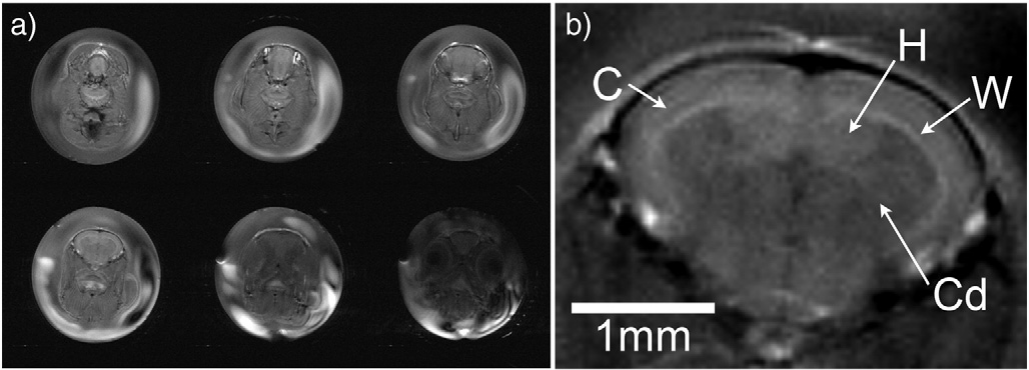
High resolution T_2_ weighted RARE brain MRI, in-plane resolution 39 μm and slice thickness 300 μm. Panel a) shows the 6 coronal slices acquired. b) Shows zoomed-in T_2_ weight image of the brain, with anatomical structures visible; C = cortex, W = white matter tract, H = hippocampus, Cd = Caudate; scale bar = 1 mm.
